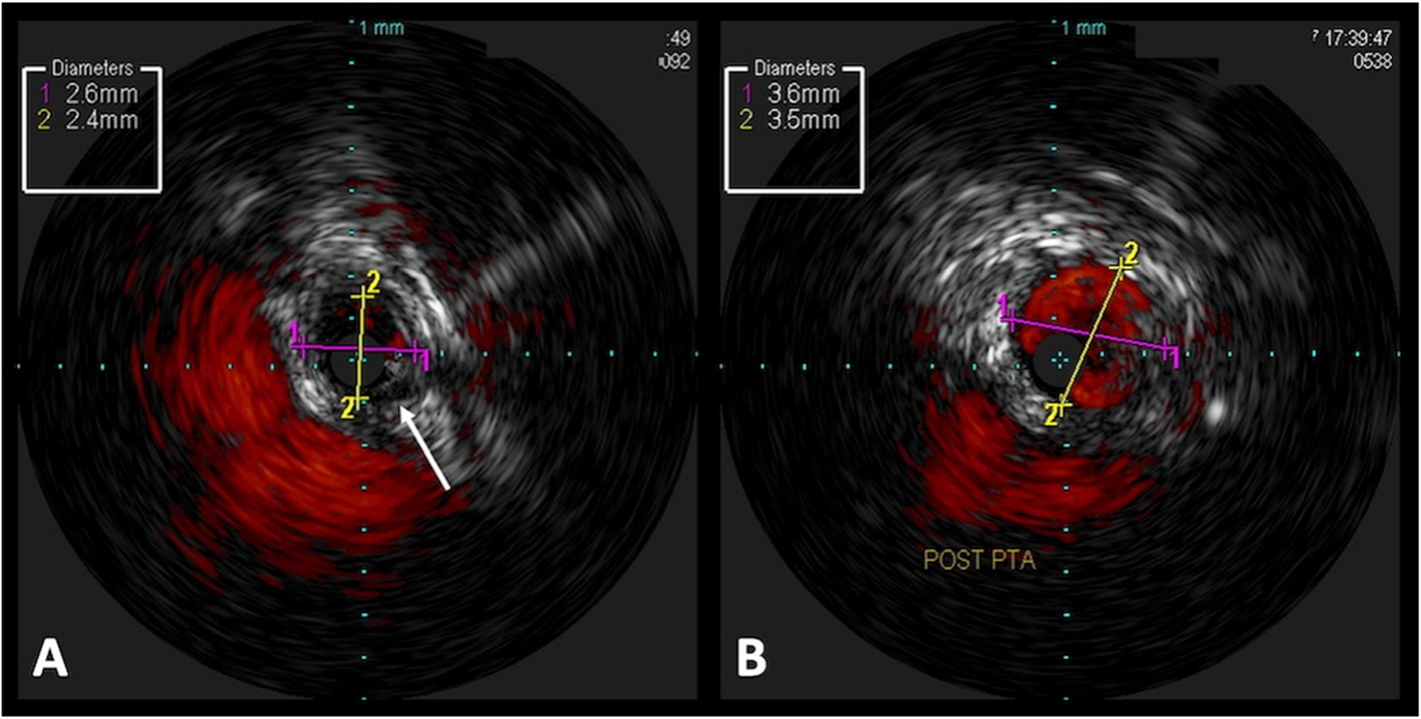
Intravascular ultrasound. a IVUS image of severe right renal artery origin stenosis with mural thickening consistent with FMD. b Post angioplasty IVUS image of improved flow and vessel diameter right renal artery origin
